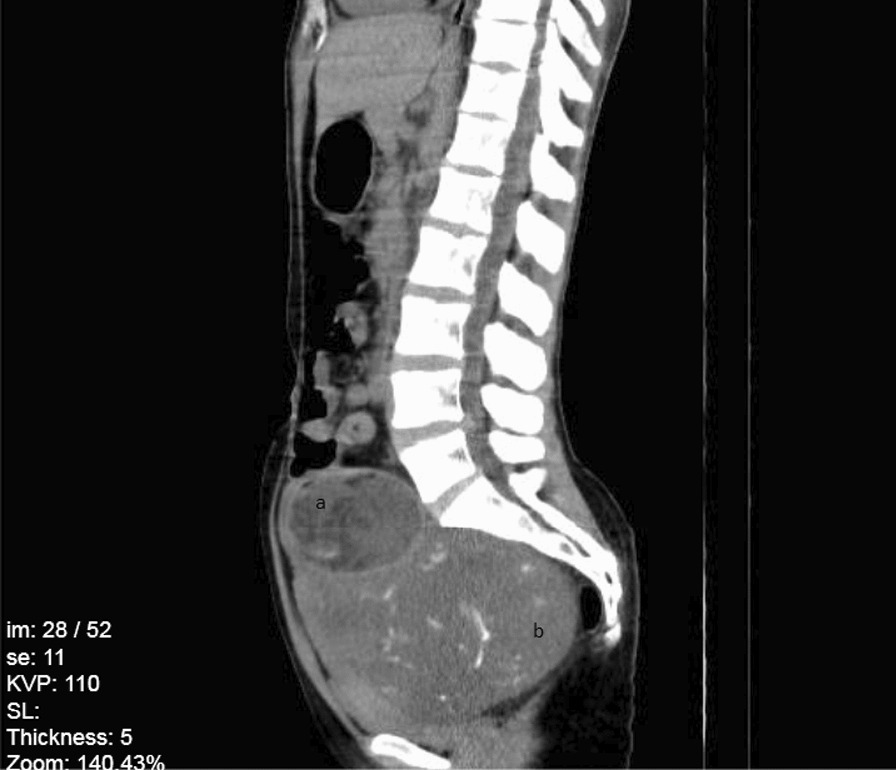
Abdominal CT-Scan without IV contrast, sagittal view. a Uterine, b Left Ovarian mass
